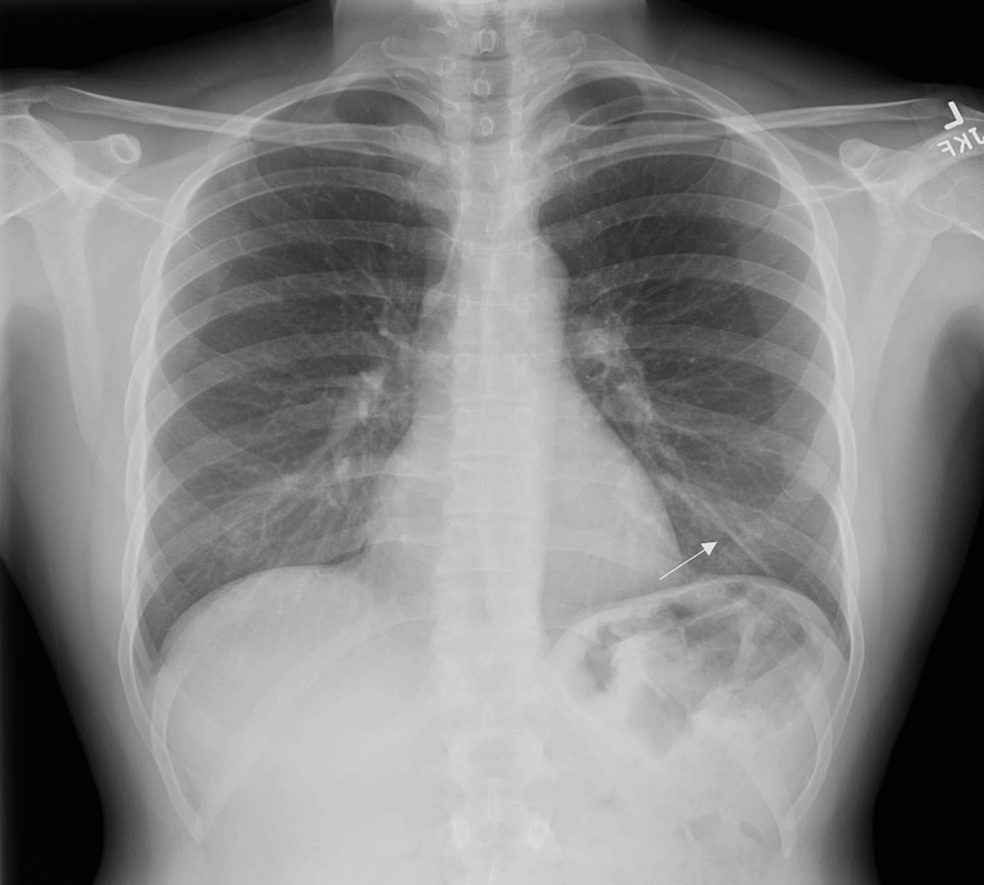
Chest X-ray showing implant in the left lung (arrow).
